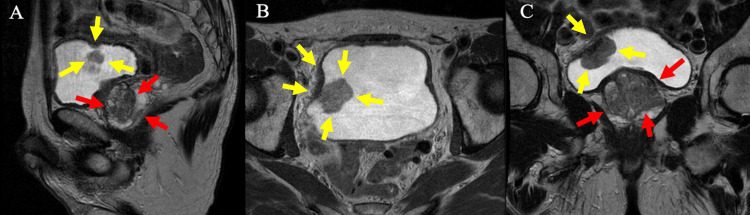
Multiparametric prostate magnetic resonance imaging (mpMRI) of the prostate showing prostate and bladder masses A. sagittal, B. axial, C. coronal; images of a mpMRI of the prostate (T2-weighted sequence). Yellow arrows indicate bladder tumor and red arrows indicate suspected prostate cancer.
